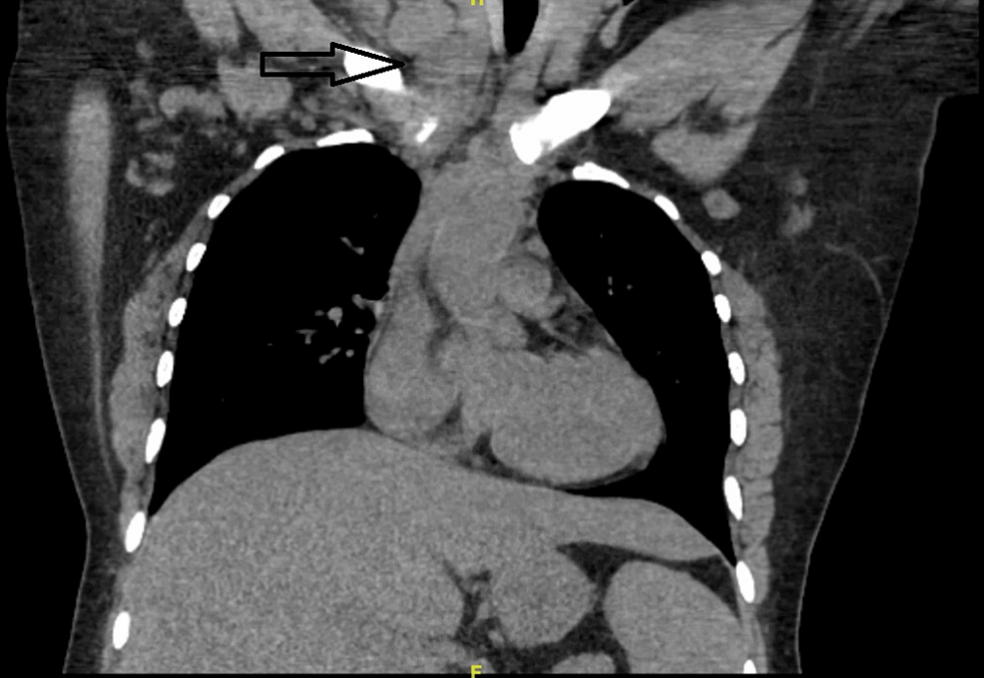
CT chest without contrast demonstrating prominent bulky lymphadenopathy in the supraclavicular regions. CT, computed tomography
